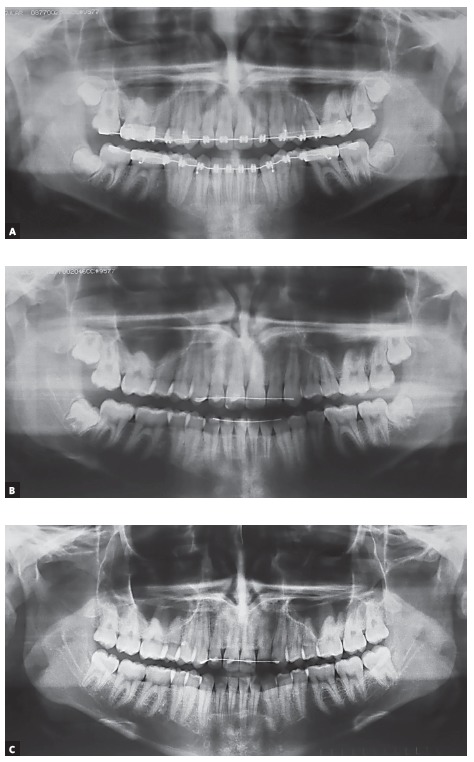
Patient aged 14 years and 6 months old at orthodontic treatment completion (A). When examining this radiograph, 52% of oromaxillofacial surgeons and 37% of orthodontists indicated tooth #48 extraction. Extraction of tooth #38 was indicated by 44% of surgeons and 48% of orthodontists. Two years later, another panoramic radiograph (B) was used to follow up eruption of the third molar at risk of impaction. At age of 19 years and 3 months, after follow-up of almost five years, all third molars had erupted spontaneously (C).
